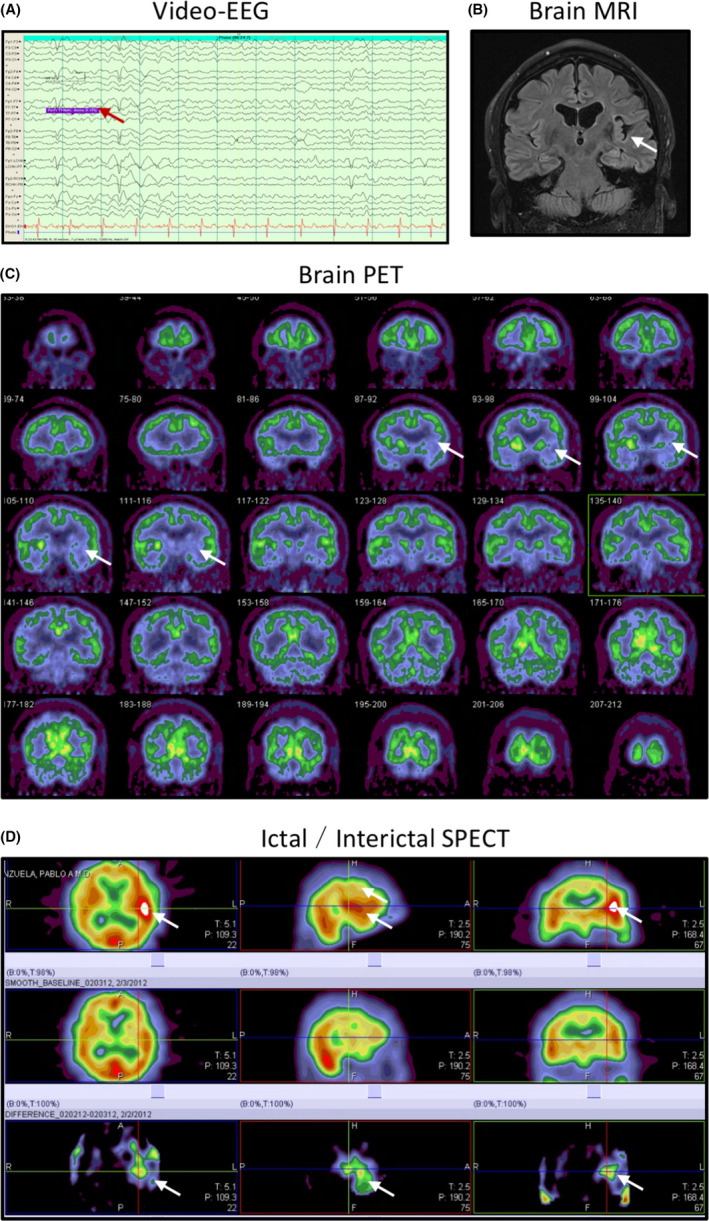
Pre‐operative evaluation of the patient for localizing the epileptogenic focus. (A) A typical seizure event on video‐EEG with left hemispheric diffuse onset and evolution. (B) Encephalomalacia in the left insula revealed by brain MRI. (C) Hypometabolism in the left insula revealed by PET scan of the brain. (D) Hyperperfusion in the region of MRI‐defined encephalomalacia in left insula after an ictal SPECT injection during a typical complex partial seizure
